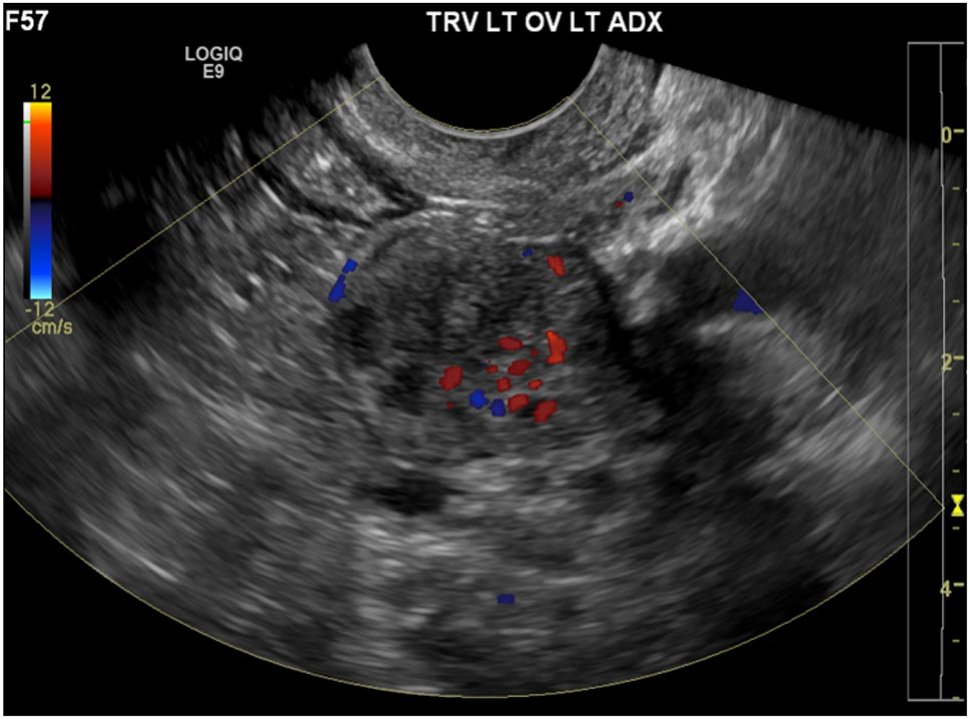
A 57-year-old woman underwent pelvic ultrasound exam showing a solid left ovarian mass with internal vascularity on Doppler ultrasound exam
